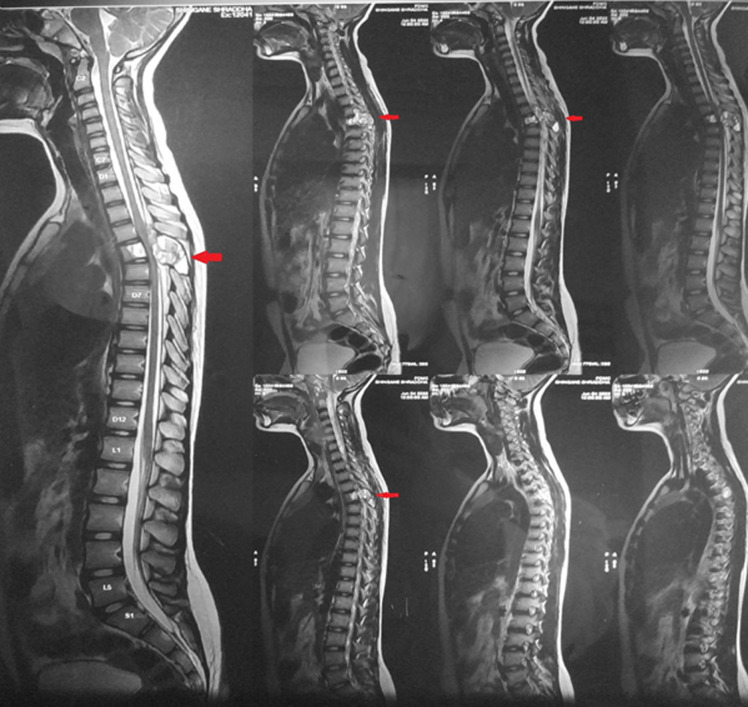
MRI finding of aneurysmal bone cyst at D4-D5 level
